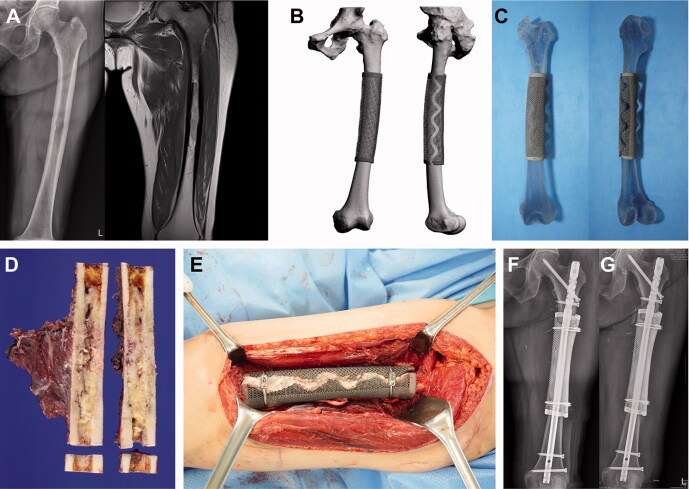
Femoral reconstruction. Images of patient #9. (A) Preoperative plain radiographs and a T2-weighted MR image showing metastatic renal cell carcinoma arising from the femoral shaft. (B) Graphical designs and (C) photograph of the 3D-printed implant. (D) Resected bone tumor as planned. (E) Intraoperative photograph and (F) postoperative plain radiograph showing femoral reconstruction. (G) Follow-up plain radiograph at 3 months postoperatively showing callus formation at both proximal and distal junctions.
